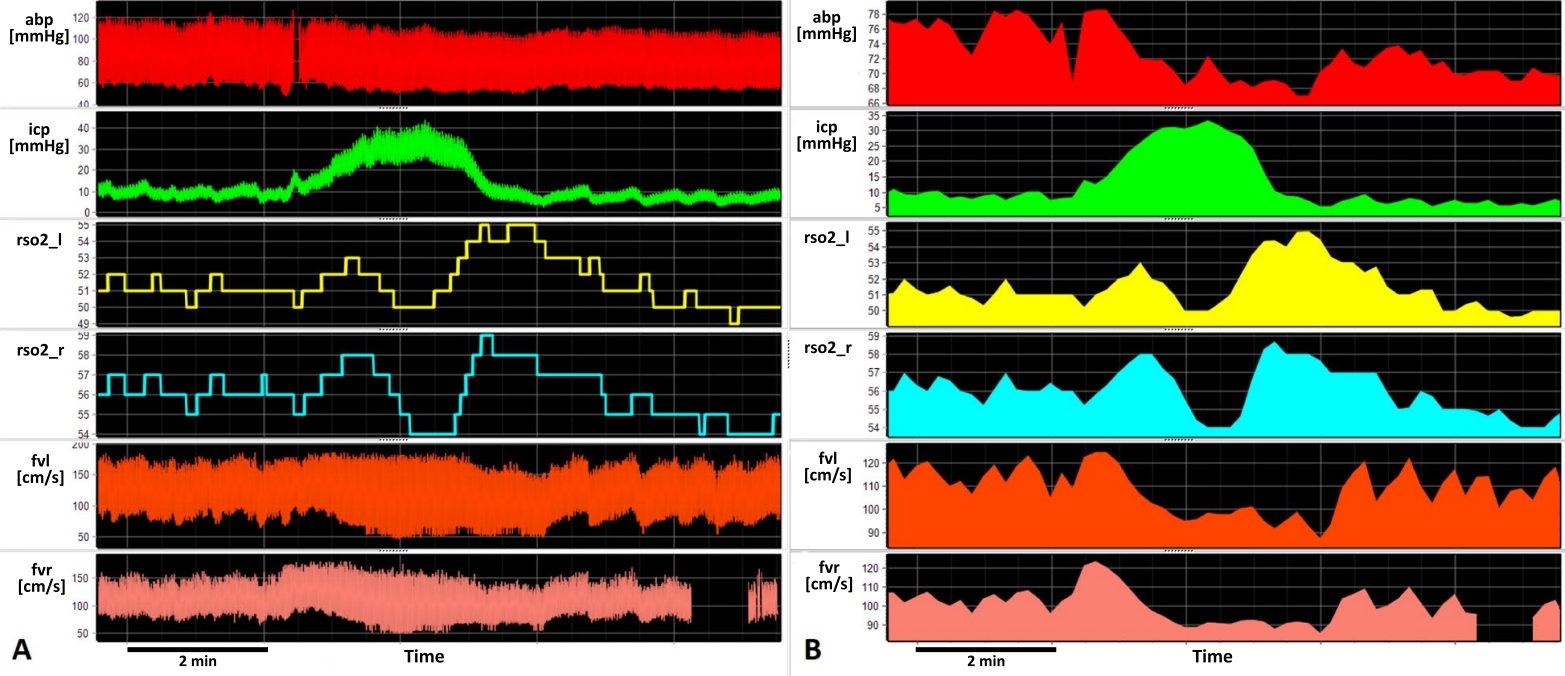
Plateau wave in a TBI patient. abp: arterial blood pressure; icp: intracranial pressure; rso2_l: brain oxygen saturation collected with near infrared spectroscopy, left side; rso2_r: brain oxygen saturation, right side; fvl: flow velocity left acquired with transcranial doppler; fvr: flow velocity right. On the left (A) raw signals collected during multimodal monitoring in ICU. On the right side, the same raw signals are represented as means over 10 s. While ICP increases, it is possible to see that flow velocity decreases in both sides, as well as the brain regional oxygen saturation
